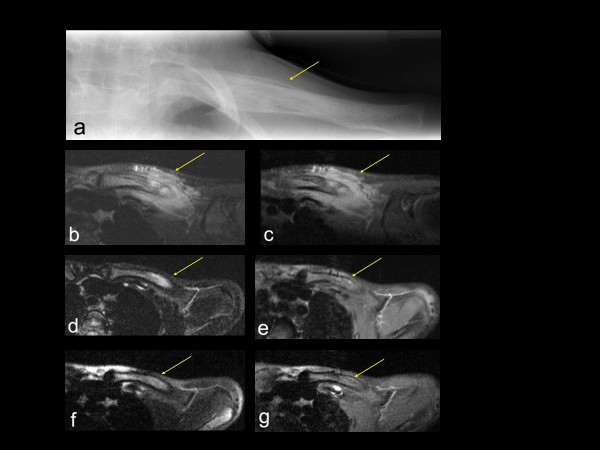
Imaging data of CRMO lesion involving left clavicle in a 7-year old girl (A). Pre-treatment imaging. Plain radiograph of the left clavicle demonstrates periosteal new bone formation (arrow). (B-C). Pre-treatment MRI: (B) Axial (fat-saturated, T2-weighted) and (C) post gadolinium MRI: Hyper-intense T2 signal with post-contrast enhancement is seen within the clavicle (arrow) with marked soft tissue inflammation (arrow). (D-E). Post-treatment MRI (5 months after initiation of treatment with pamidronate) with the same technique as B and C, respectively. The intra-osseous abnormal signal has significantly improved, and marked soft tissue abnormality has almost completely resolved. (F-G). Post-treatment MRI (8 months after initiation of treatment with pamidronate) with the same technique as B and C, respectively, reveals complete resolution of the intra-osseous abnormal signal.
